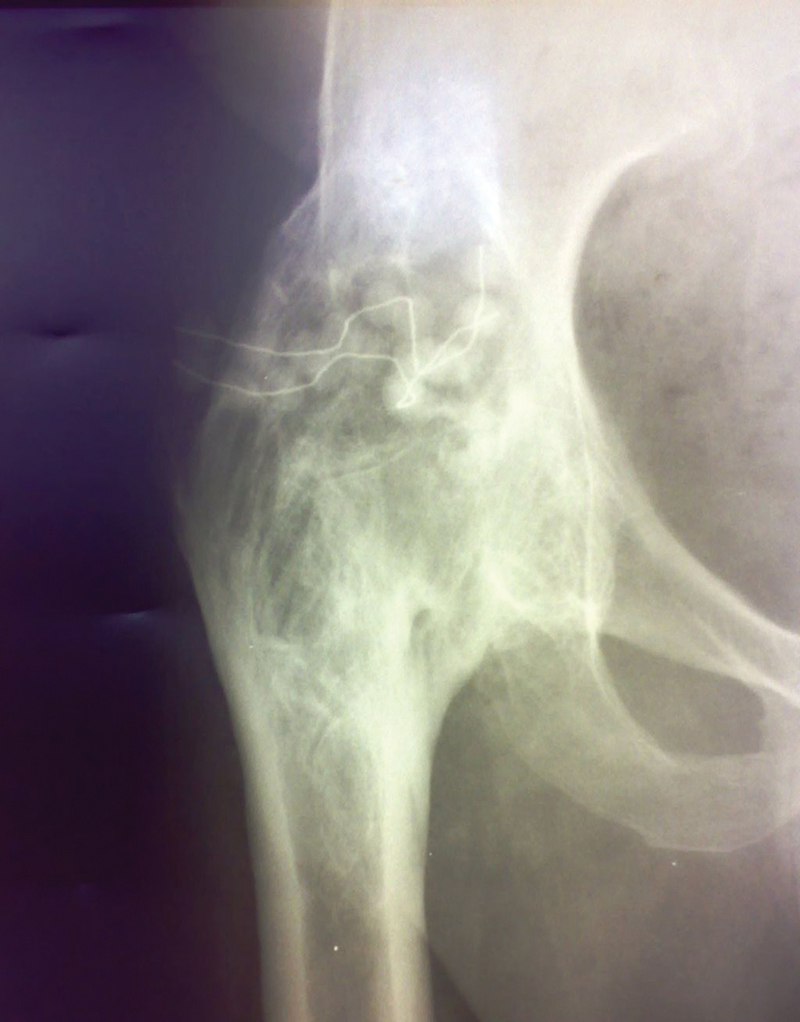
A plain radiograph of the right hip showing the containment of the gentamicin beads.
